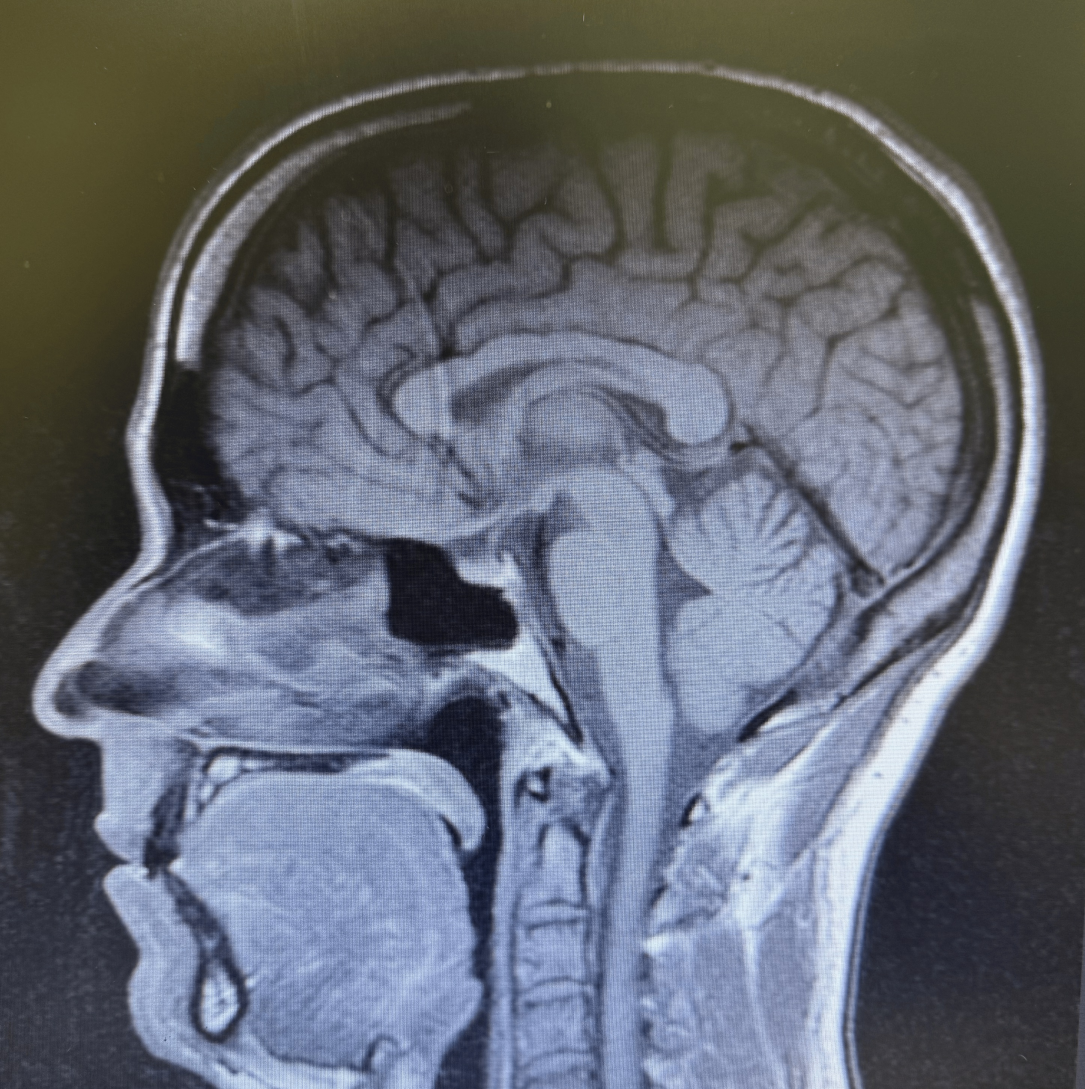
Magnetic resonance imaging (MRI) of the brain sagittal view showing normal findings
